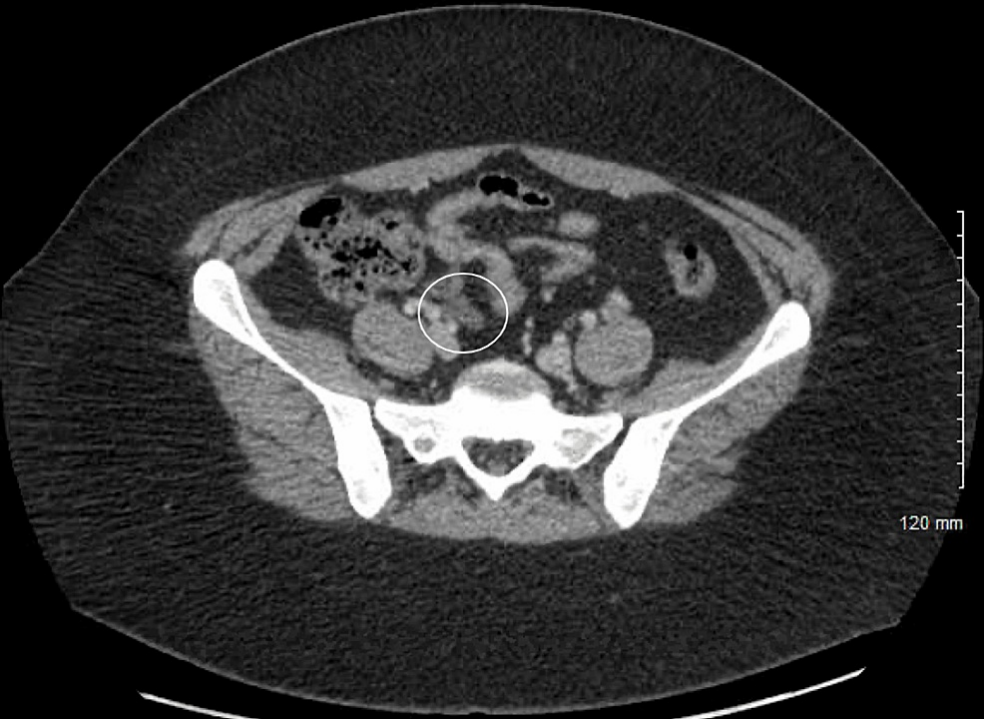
Admission CT scan Axial CT with IV contrast demonstrates a mildly dilated appendix encircled above. CT: computed tomography; IV: intravenous
